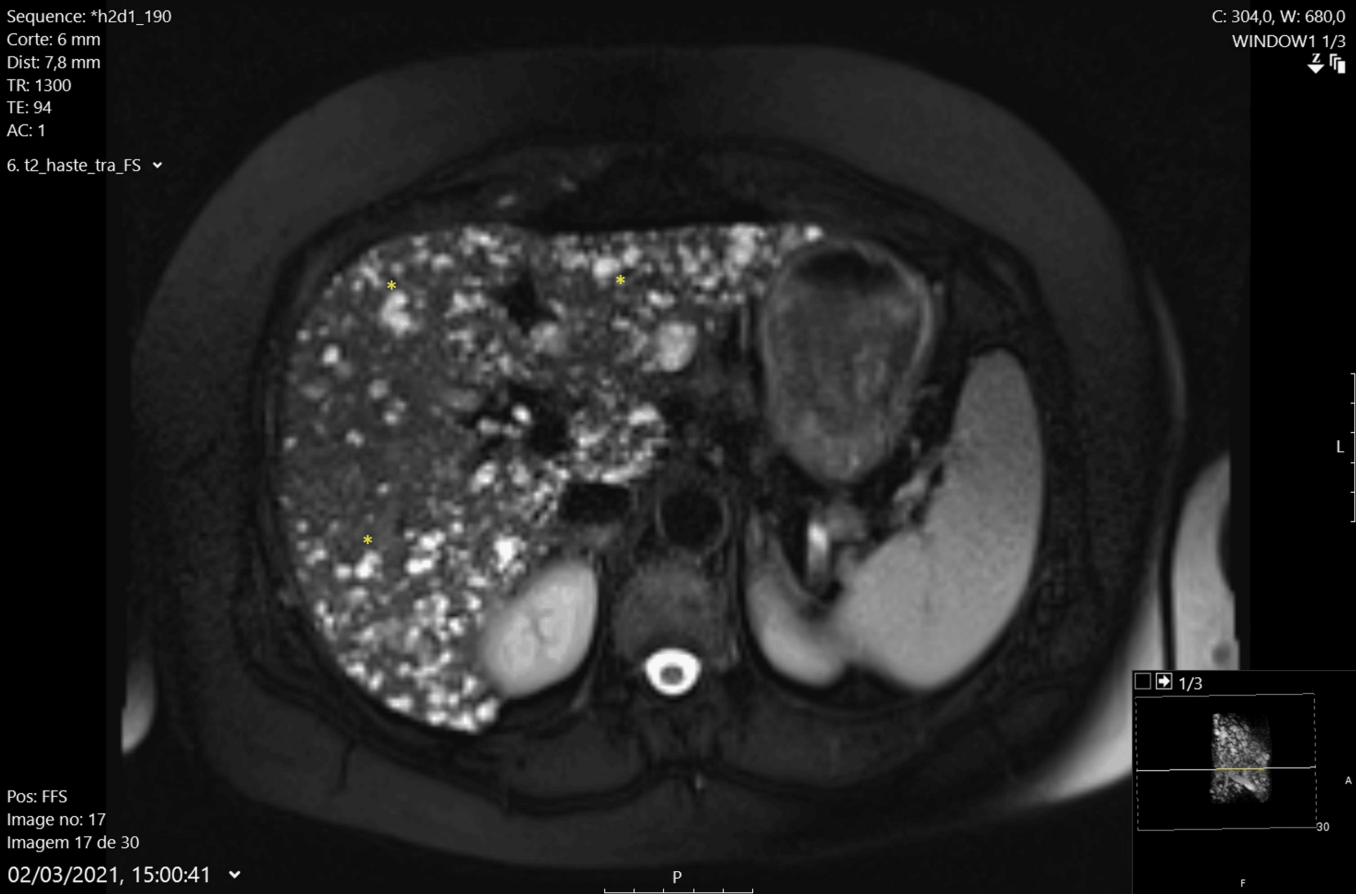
Axial MRI View of T2 Hyperintense Foci Axial MRI view displaying numerous T2 hyperintense lesions within the liver, indicative of Von-Meyenburg complexes (*). The image demonstrates the extensive distribution of these benign biliary hamartomas (*).
